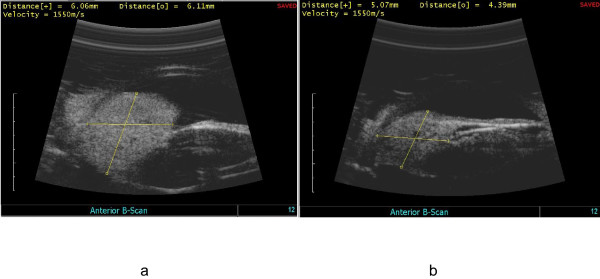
(a) High-frequency immersion B-scan ultrasonography of the right eye reveals the ciliary body mass at a peak size of 6.11 × 6.06 mm. (b) B-scan ultrasonography after proton radiotherapy reveals the regression of the ciliary body mass size to 5.07 × 4.39 mm.
